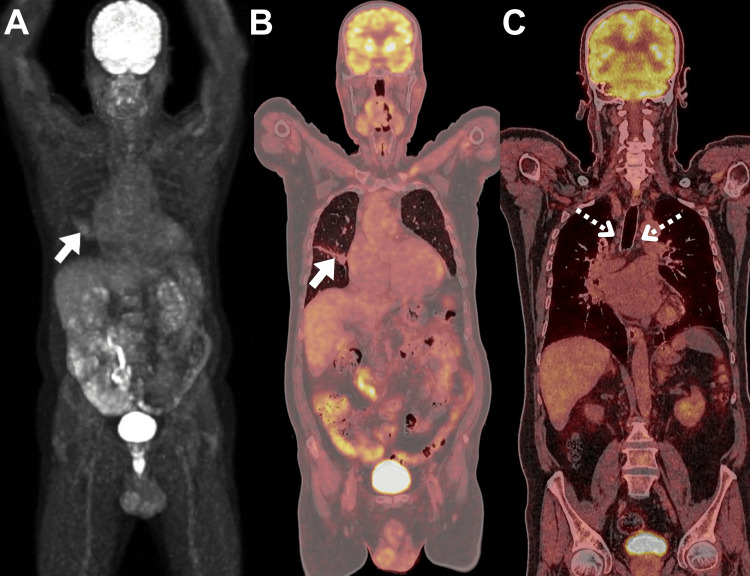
18F-FDG PET/CT images of the patient. (A) MIP, and (B, C) coronal views of 18F-FDG PET/CT images obtained for initial staging. The scan revealed evidence of a mildly hypermetabolic right pulmonary mass (SUVmax of 2.8; arrows), comparable to that of the liver reference. This was associated with multiple non-18F-FDG-avid mediastinal lymph nodes (SUVmax of 1.7; dotted arrows). MIP: maximal intensity projection; 18F-FDG PET/CT: fluorine 18-flurodeoxyglucose positron emission tomography/computed tomography; SUV_max_: maximum standardized uptake value.
